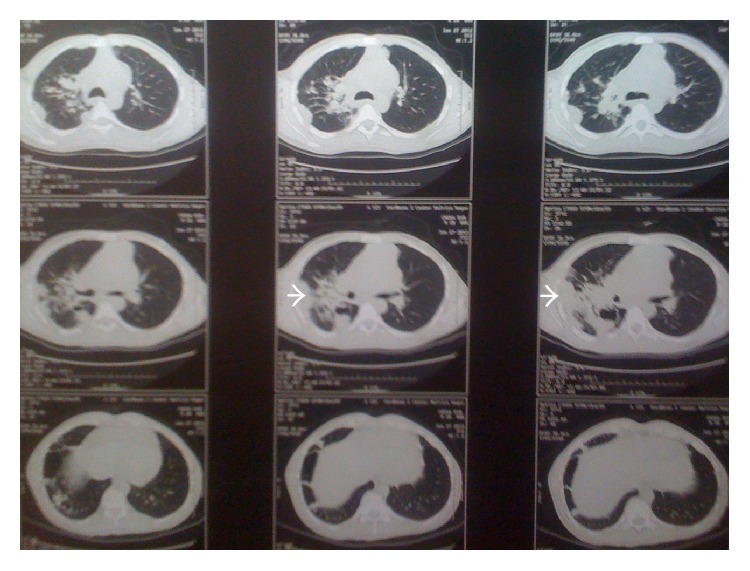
CT scan of the chest showing lesion in the lungs. Biopsy of these lesions was inconclusive.
